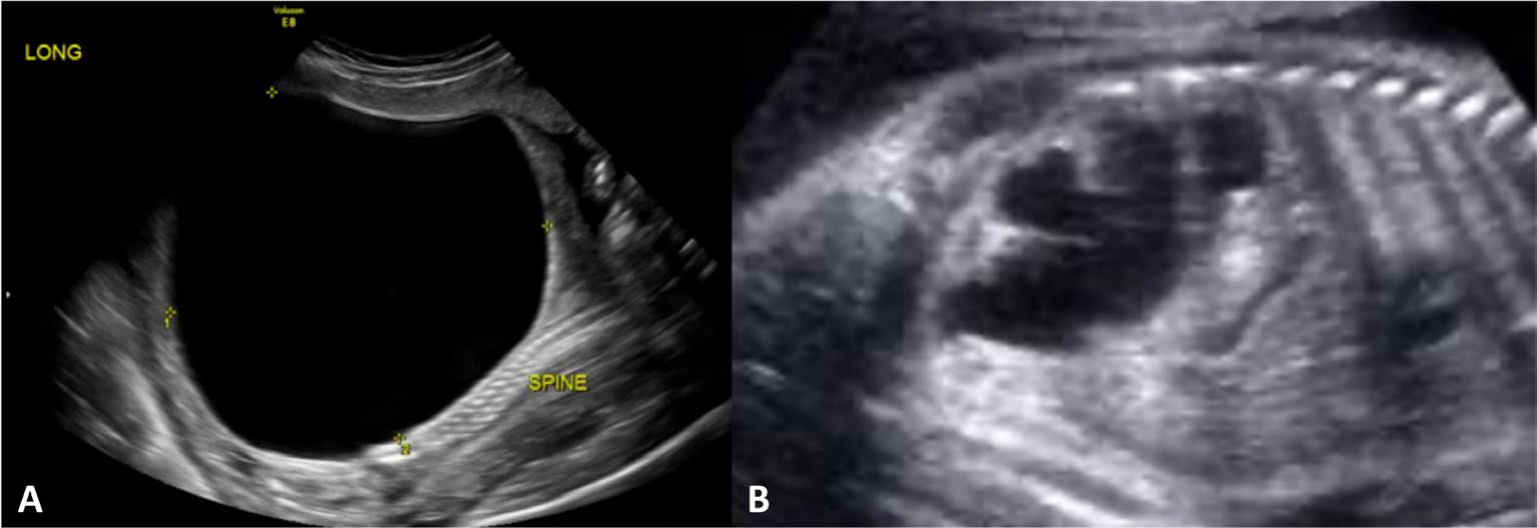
Prenatal ultrasound findings in Case 2. (A) Severely distended fetal bladder with thickened wall and “keyhole sign” at 19 weeks' gestation. (B) Bilateral hydronephrosis with normal renal echogenicity and absence of cortical cysts.
